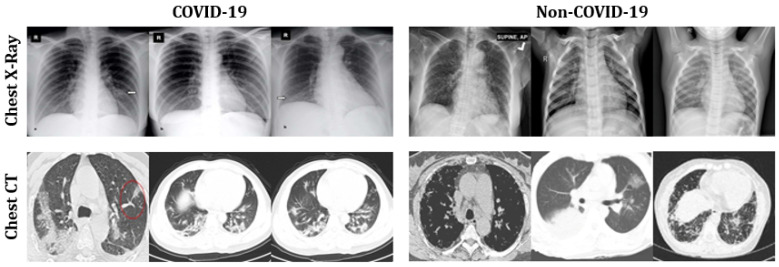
Representative sample images of chest X-rays and CT scans used in the mixed dataset adopted for analysis.
